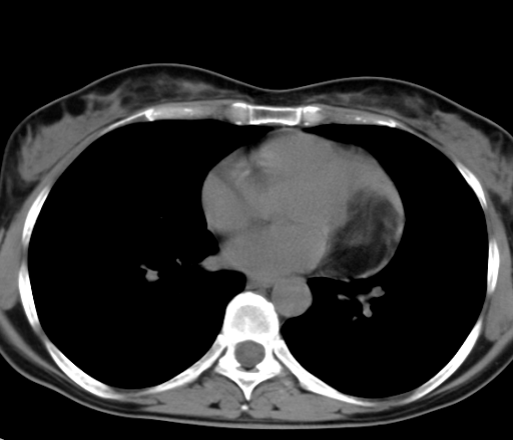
Thoracic CT scan. The large, ovoid tumor, 3cm/5cm in axial plan and 4 cm in cranio–caudal plan. Located in the intrapericardial space, along the left border of the heart, towards the base of the heart.
